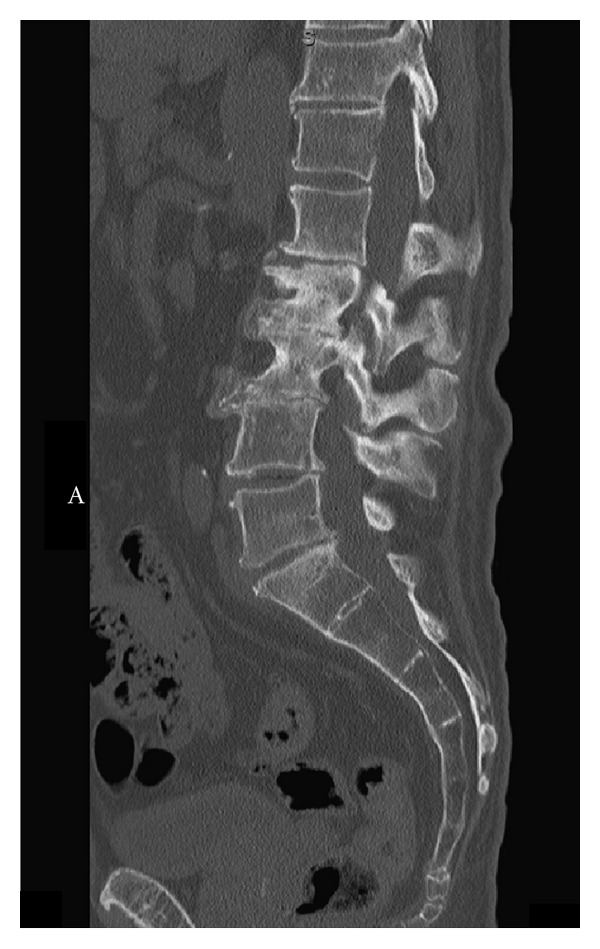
Sagittal view of the lumbar spine on CT reveals a calcified L2-L3 disc bulge with narrowing of the intervertebral disc space.
